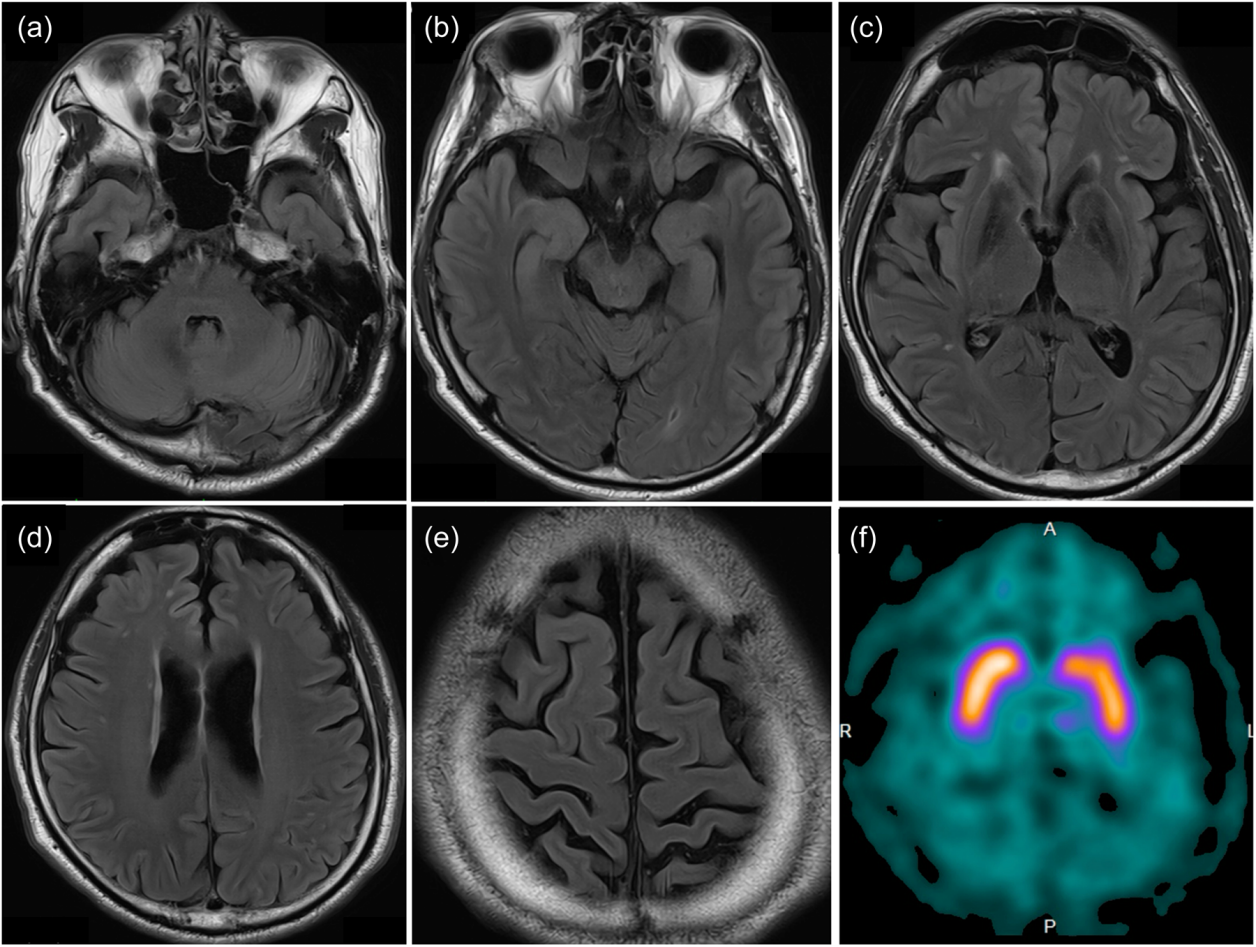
Fluid‐attenuated inversion recovery sequence brain magnetic resonance imaging showed no abnormal findings other than calcified lesions. Axial brain slices at the level of (a) pons, (b) hippocampus, (c) basal ganglia, (d) central part of lateral ventricle, and (e) central sulcus. (f) Dopamine transporter scan showed decreased in the bilateral putaminal specific binding ratios (4.43 and 4.22 for the right and left side, respectively).
